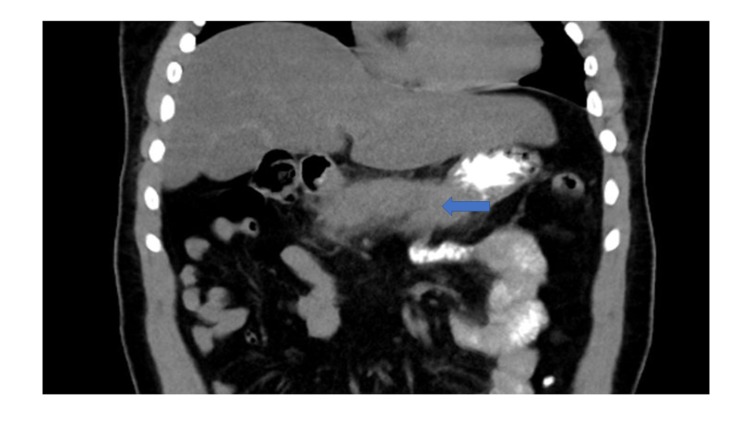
Coronal sectional image of CT abdomen with blue arrow showing the inflammation surrounding the pancreas compatible with acute pancreatitis.
